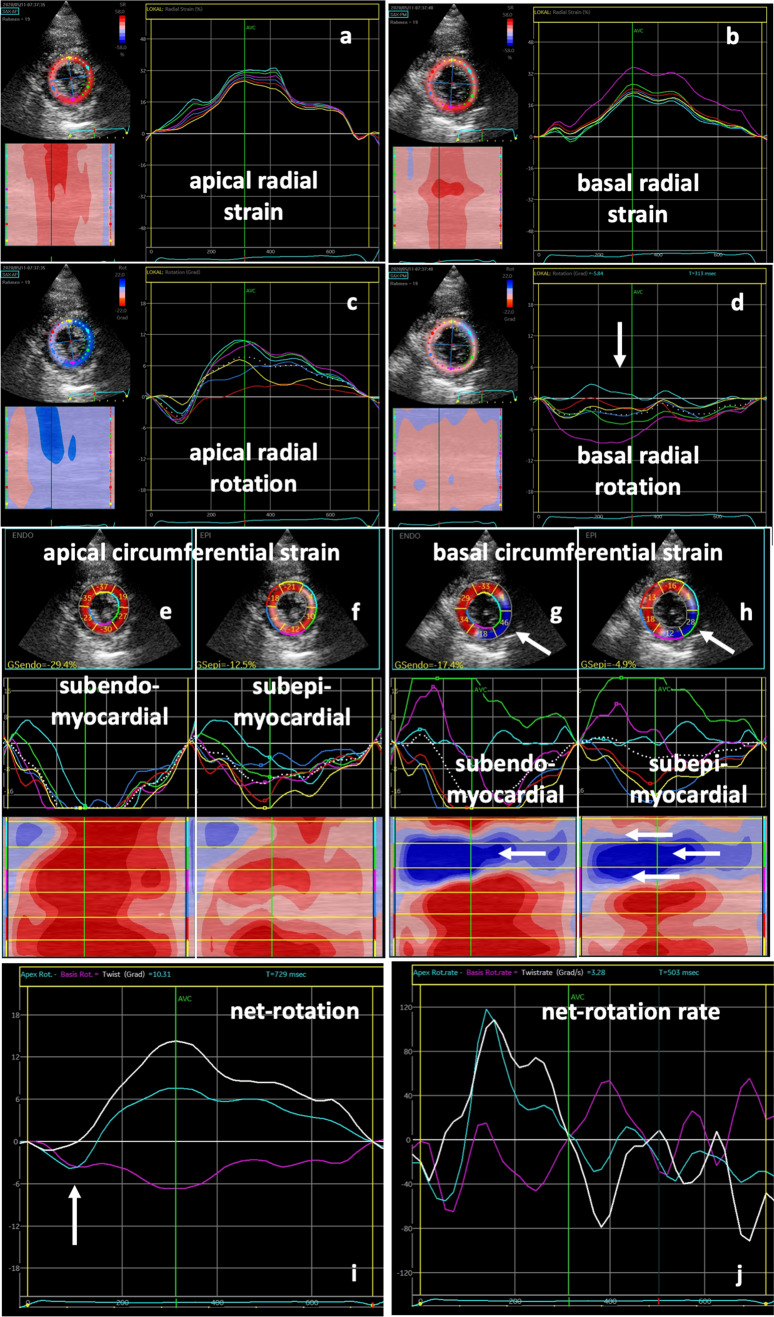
Rotational deformation pattern of the same SARS-CoV-2-infected patient with COVID-19 pulmonary disease as in Fig. 1: normal radial strain patterns are documented in apical (a) and basal (b) left-ventricular (LV) segments. Apical rotation was also normal (c), whereas basal rotation showed a regional dispersion with almost no rotation septal and anterior (d, white arrow). Below parasternal short-axis views with segmental subendomyocardial (e, g) and subepimyocardial (f, h) circumferential strain values, strain curves, and color-M-Modes are shown. Abnormal deformation is documented in basal anterior, anterolateral, and inferolateral (g, h white arrows). Line graphs of apical (blue) and basal rotation (magenta) as well as net rotation (twist) (white) (i) and corresponding line graphs of rotation rate (j) document abnormal twist (biphasic apical rotation, i white arrows) and normal untwisting (j)
